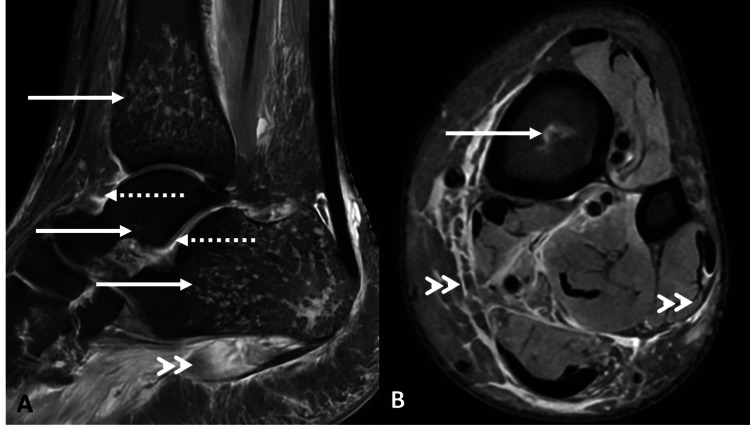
MRI of the left ankle joint A & B: PDFS sequence in sagittal (A) and axial (B) planes of the ankle joint and distal leg. Geographical areas of altered marrow signal intensities noted involving the distal aspect of tibia, calcaneum, and talus (solid white arrow). Minimal ankle joint and subtalar joint effusion (dotted white arrow). Extensive soft tissue edema surrounding the ankle joint and distal leg (double arrowhead). PDFS: proton density fat-saturated
